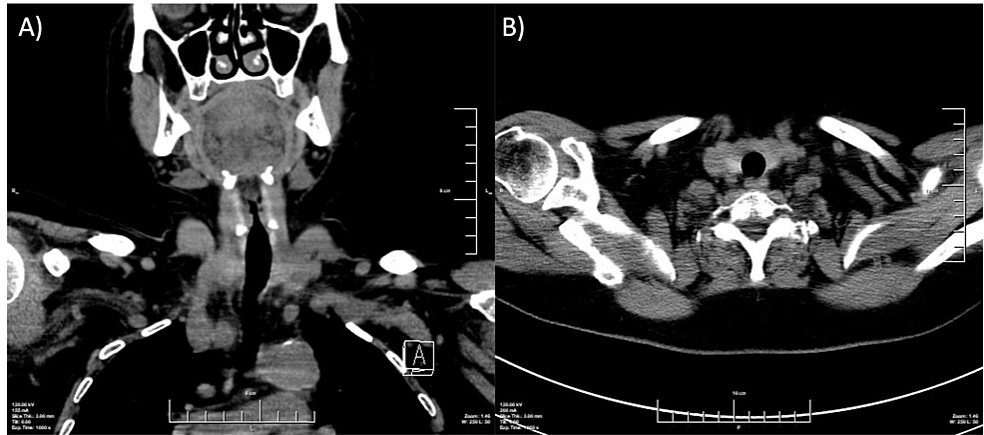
Post-chemotherapy CT scan CT image of the neck after six courses of chemotherapy. No tumor formation is present.
